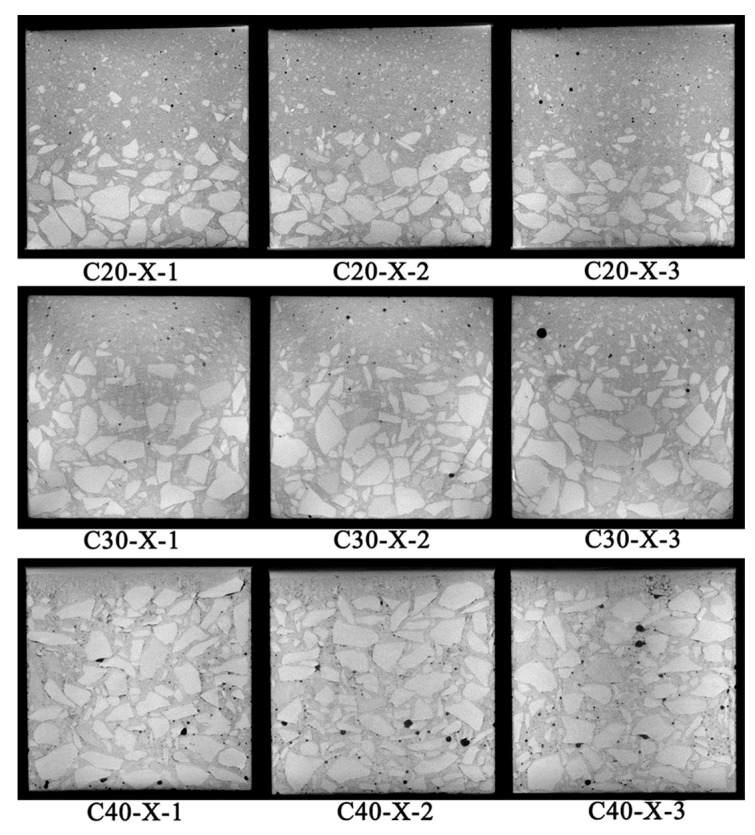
CT scans at room temperature.
